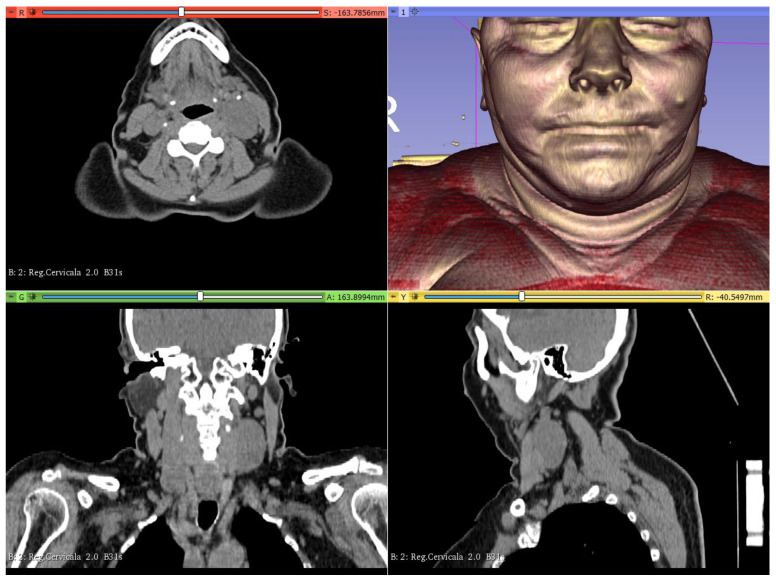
Axial view (red), coronal view (green), sagittal view (yellow), and 3D view (blue) of the head and neck computer tomography (CT) in 3D Slicer (www.3dslicer.org (accessed on 4 October 2021)) software. A well circumscribed cervical mass was observed in all sections. Asymmetric deformation of the left side of the neck was observed on the 3D view (blue).
